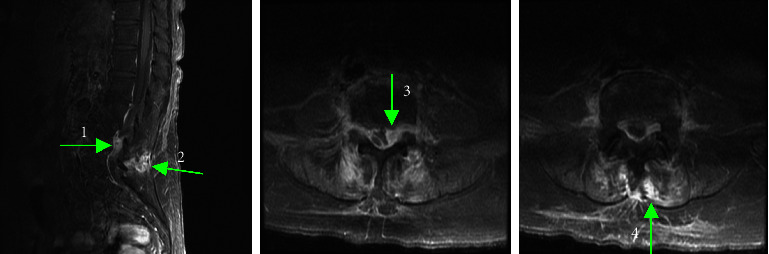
T1 sagittal and axial contrast-enhanced MRI obtained prior to surgery, showing focal, small, lobulated fluid collection at the posterior epidural space of L4-L5 (arrows 1 and 3), with adjacent soft-tissue swelling at the epidural space and posterior column of the L4-L5 level (arrows 2 and 4).
